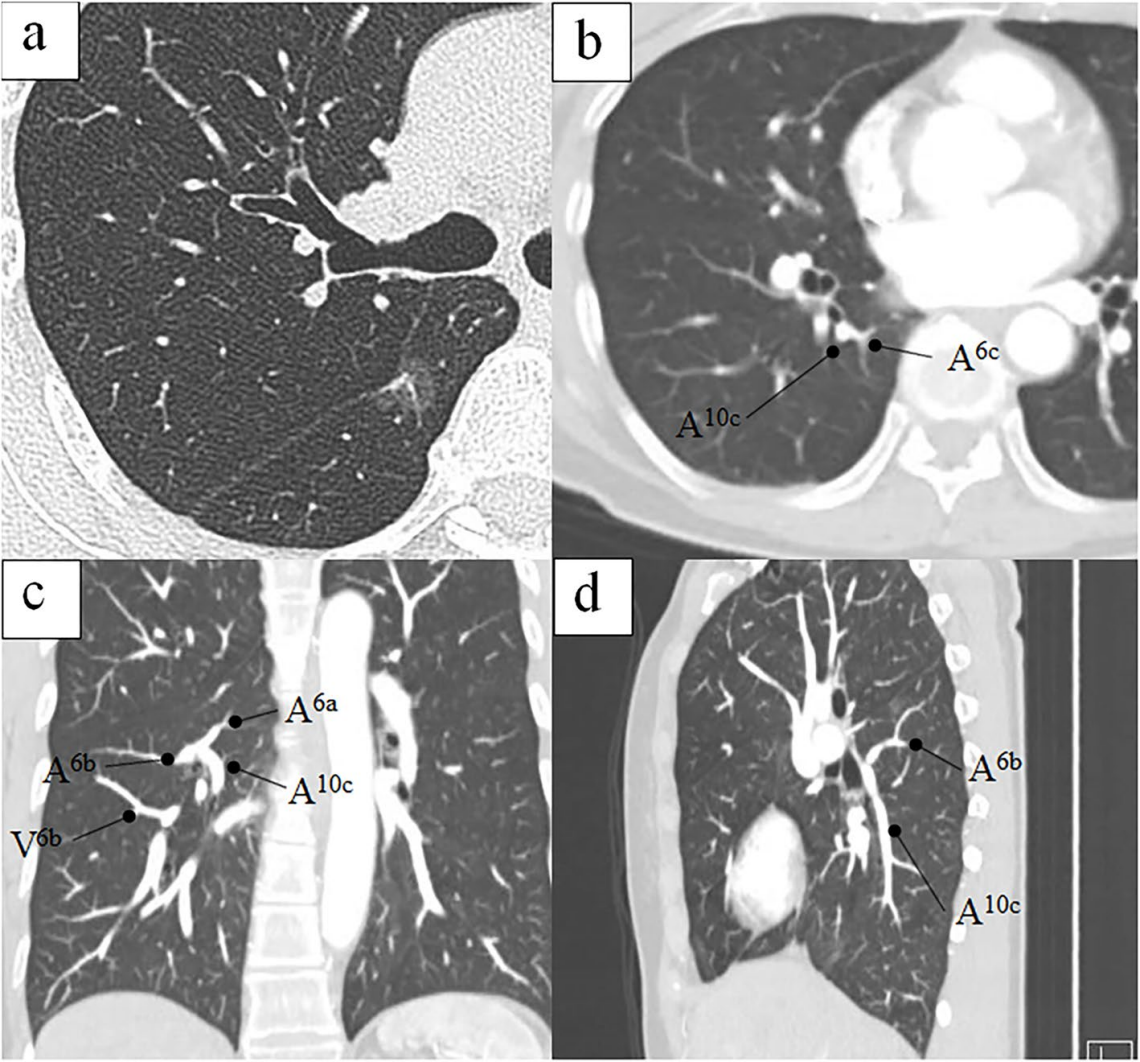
The chest computed tomography (CT) shows the tumor 14 mm in diameter and a solid component (4 mm) a. Preoperatively, multi-planar reconstruction CT shows an anomalous posterior basal pulmonary artery (A10c), branching from the right lower superior segment artery (A6) (b axial image, c coronal image, d sagittal image)
